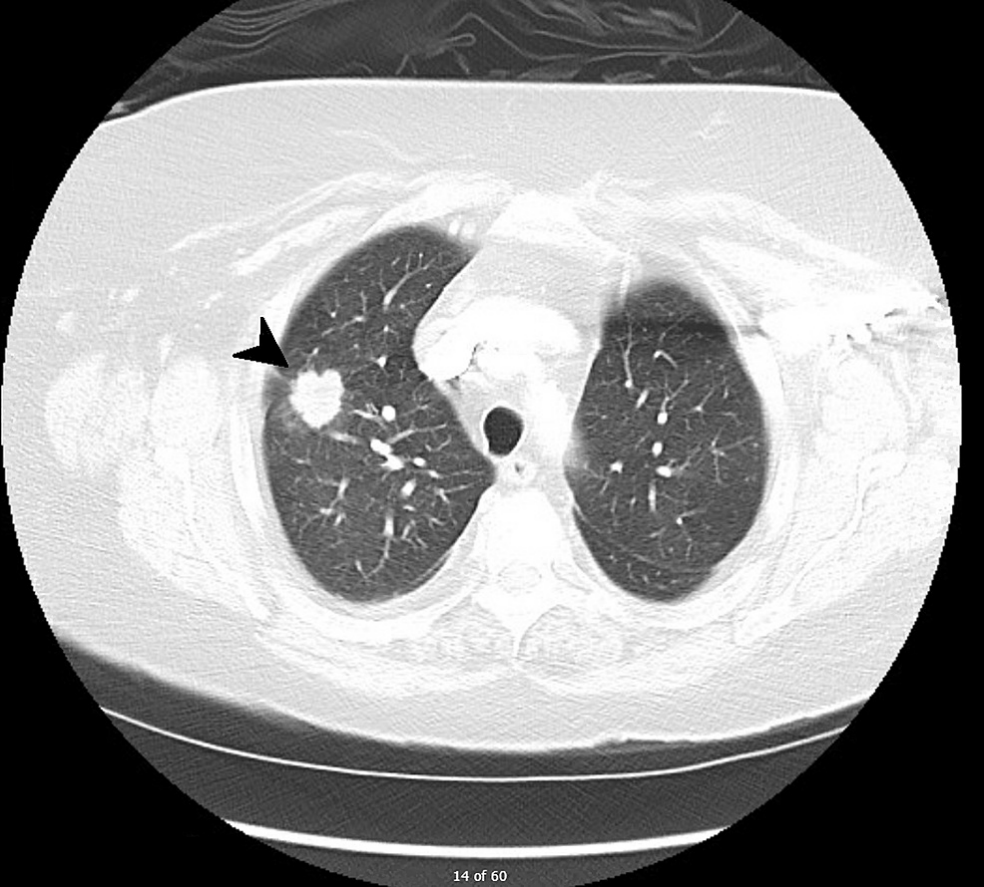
Chest CT showing an upper right lobe nodule (black arrowhead) CT: computed tomography
